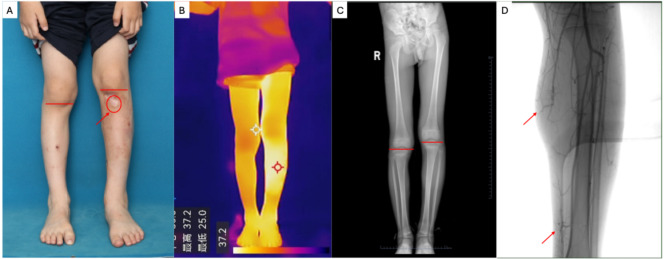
Clinical manifestation and radiograph of the patient. A: leg length discrepancy with capillary malformation. The red arrow and circle showed formal ischemia, ulcer and scar lesion. The red line showed the different knee level. B: The temperature of capillary malformation is higher than adjacent tissue and the area of the healthy leg in the thermometric indicator. C: Digital radiography of the lower extremities showed longer tibia in the lesional leg than the healthy one
